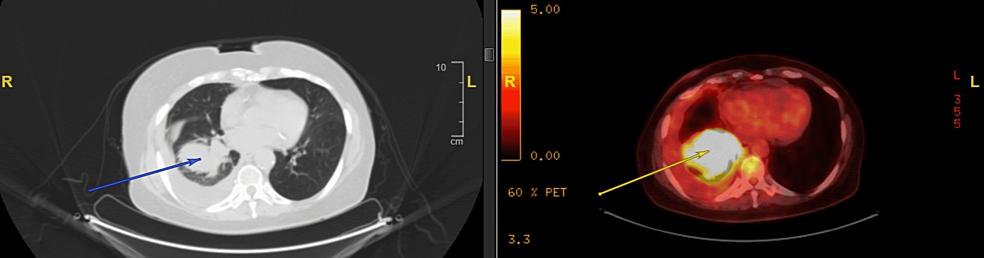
PET-CT scan axial section demonstrating a metabolically active right lower lobe heterogeneous mass (arrows) and right pleural effusion PET: positron emission tomography
